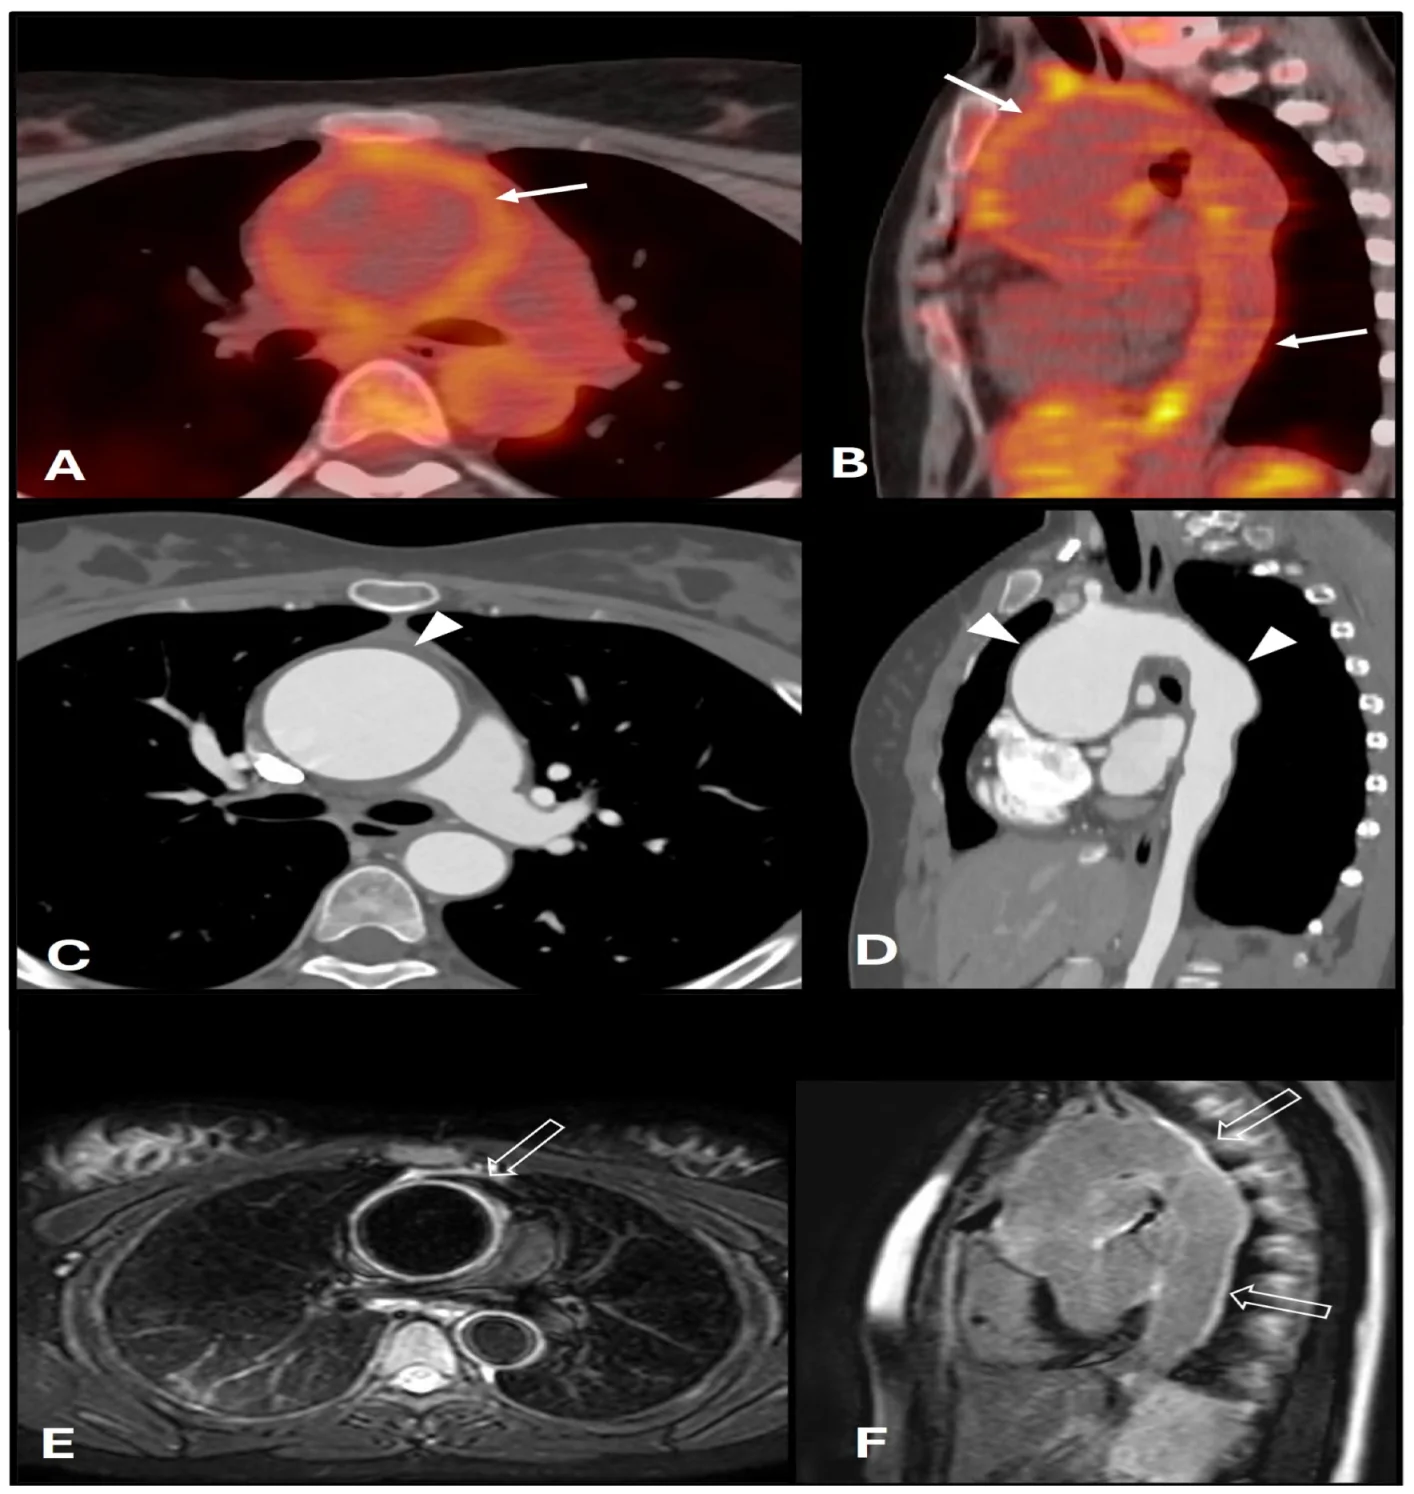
Takayasu’s disease with aortic involvement and thoracic aorta aneurysm off therapy evaluated for disease activity. Axial (A) and sagittal (B) fused images of F-18 FDG PET/CT showing extensive pathological tracer activity throughout a dilated thoracic aorta (arrows) involving predominantly the ascending aorta to a lesser extent arch and descending aorta most consistent with active vasculitis. Axial (C) and sagittal (D) images of CTA of the chest with IV contrast showing aneurysmal dilation to the ascending and to a lesser extent descending aorta associated to diffuse mural thickening (arrowheads). Axial STIR (E) images of chest MR showing diffuse aortic wall edema (open arrow). Sagittal double inversion recovery images after IV gadolinium administration (F) of chest MR showing diffuse thoracic aorta wall hyperenhancement (open arrow) consistent with active inflammation.
